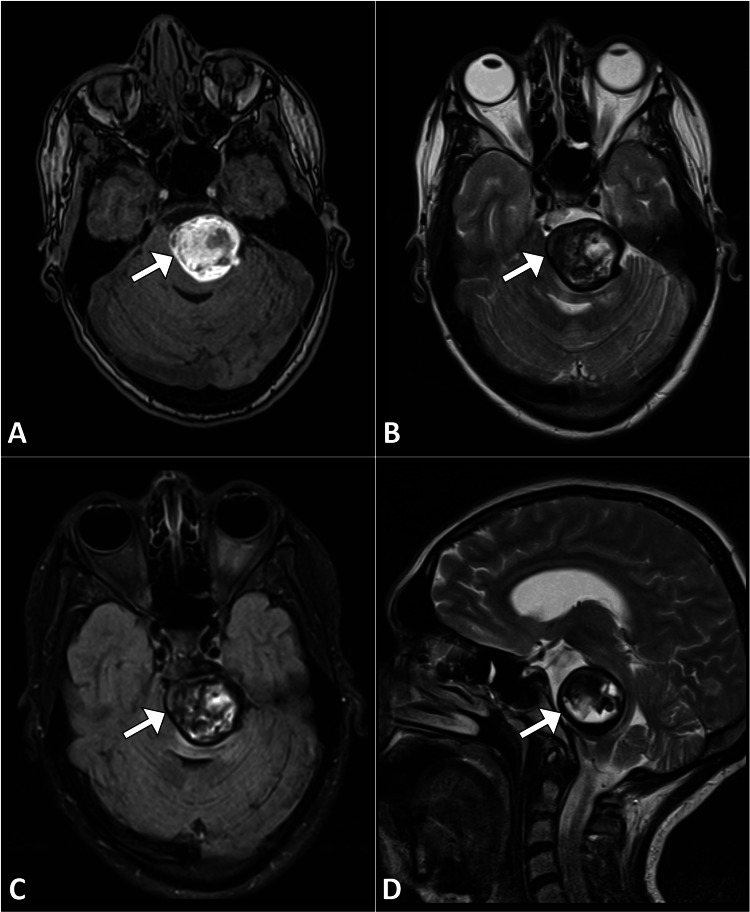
Axial (A-C) and sagittal (D) MR images depict the basilar artery's aneurysmal dilatation (arrow) with partial thrombosis, as observed through T1-weighted image (A), T2-weighted image (B), and FLAIR sequence (C) MR: magnetic resonance; FLAIR: fluid-attenuated inversion recovery
